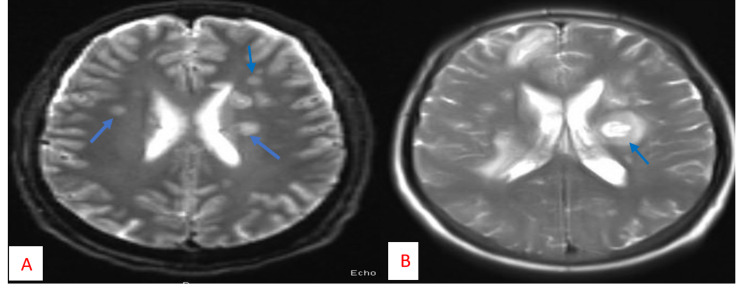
MRI of the head A. First T1Wi MRI head showing bilateral cerebral abscess. B. Repeat T1Wi MRI head 20 days after the first MRI showing new lesions MRI: magnetic resonance imaging
